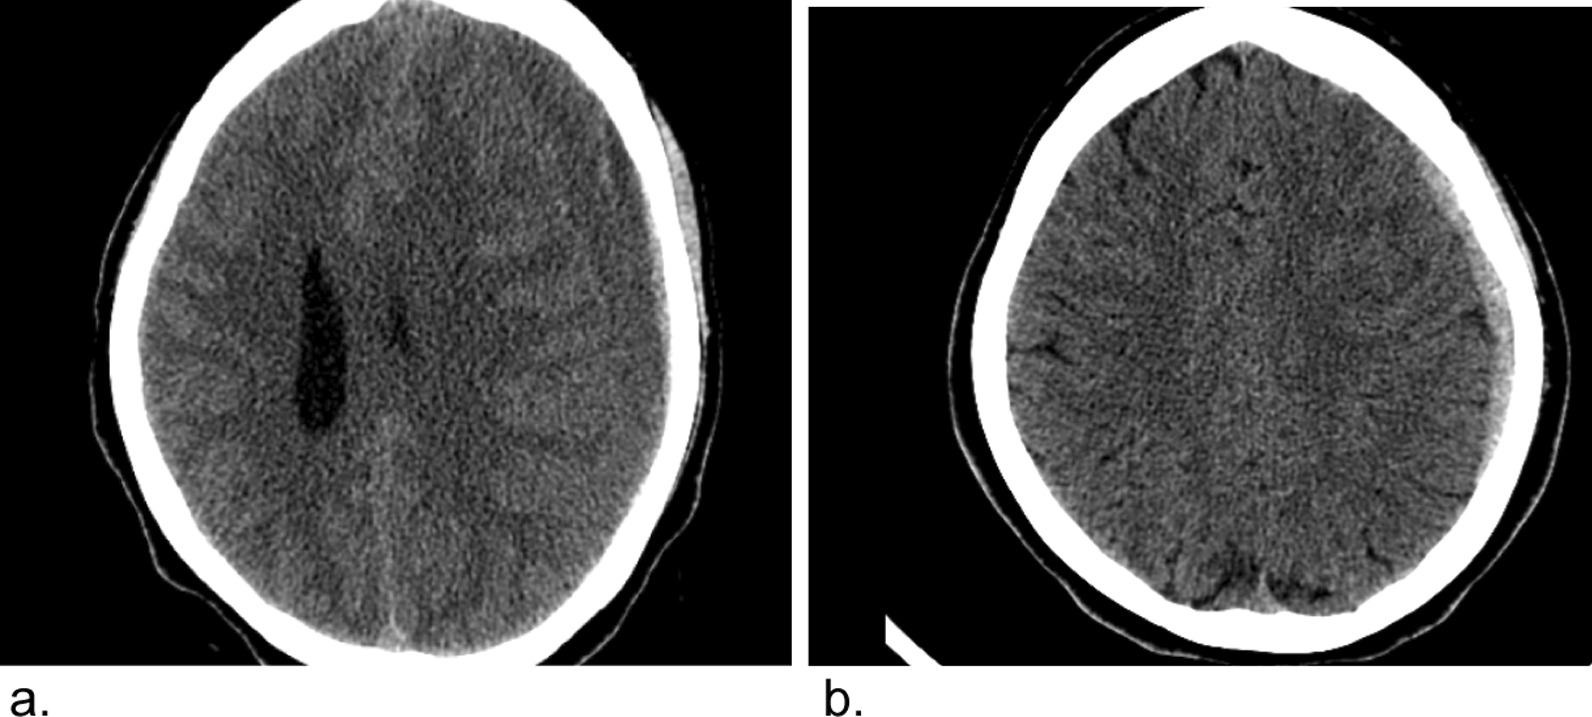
Pre- and postoperation Axial precontrast CT scan: a large left frontoparietal chronic subdural hematoma with areas of acute bleeds (a) that shows significant improvement after operation (b)
